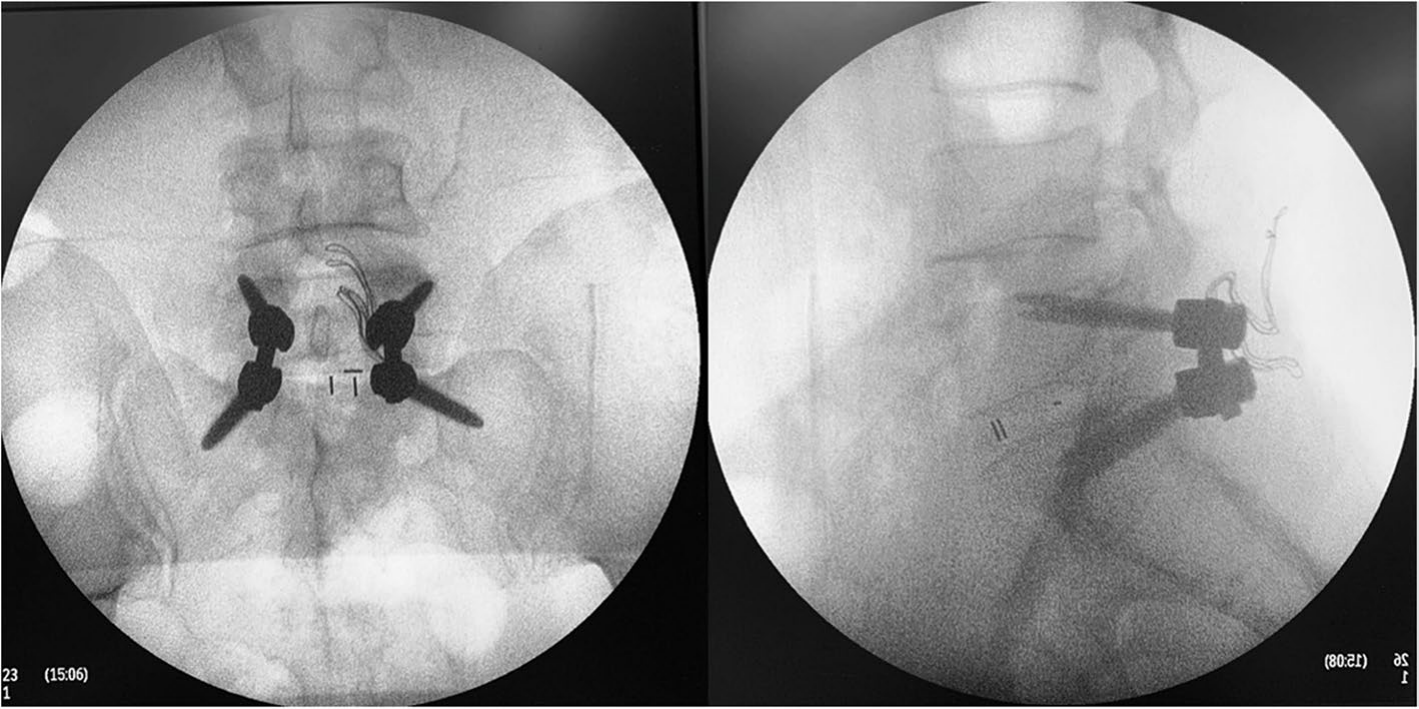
Intraoperative radiographs of L5/S1 LP-PLSIF surgery. L5 is fixed with CBTS and S1 is fixed with SAS
